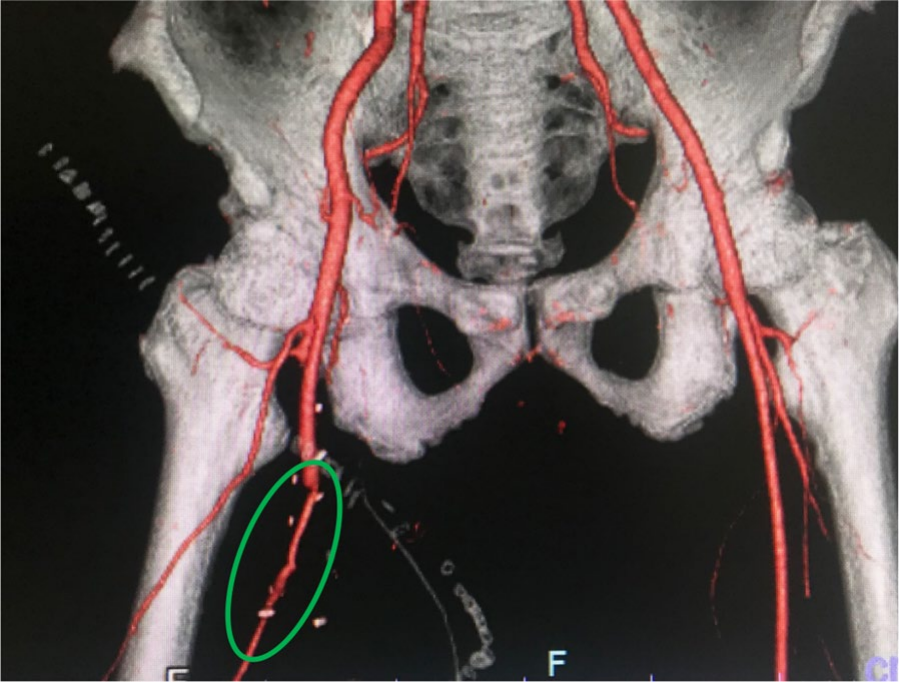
Contrast-enhanced computed tomography image obtained 8 days after surgery showed the patency of blood flow through the reconstructed femoral vessels (green oval)
